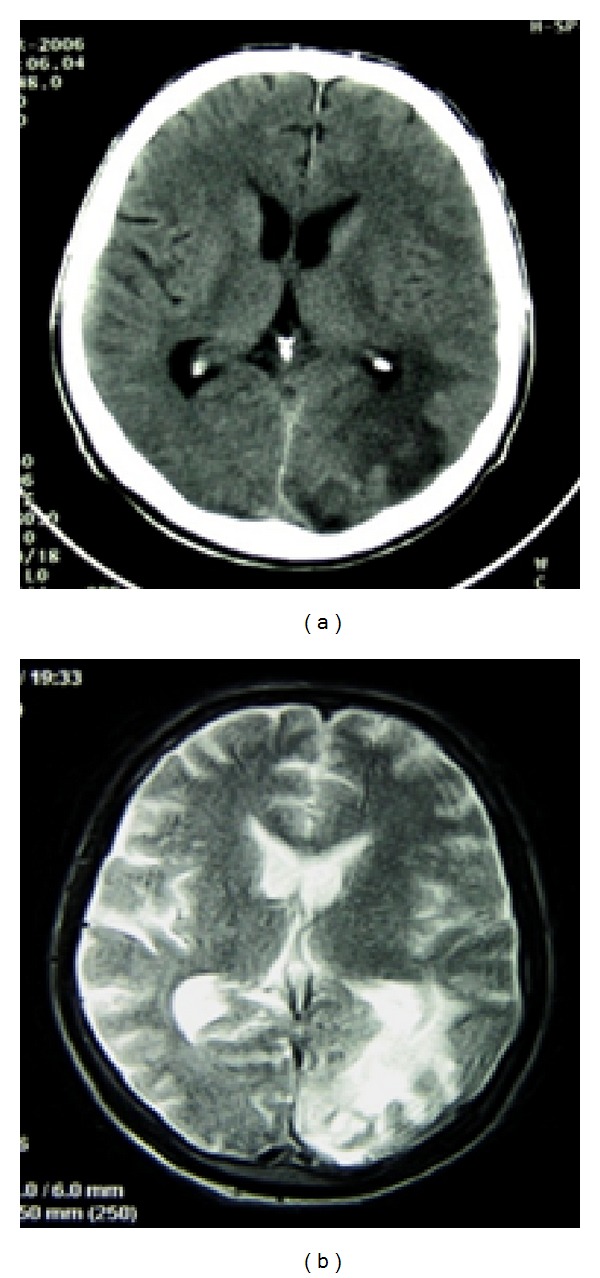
Metastatic changes in the left occipital region—a CT image (a) and an MR image (b).
